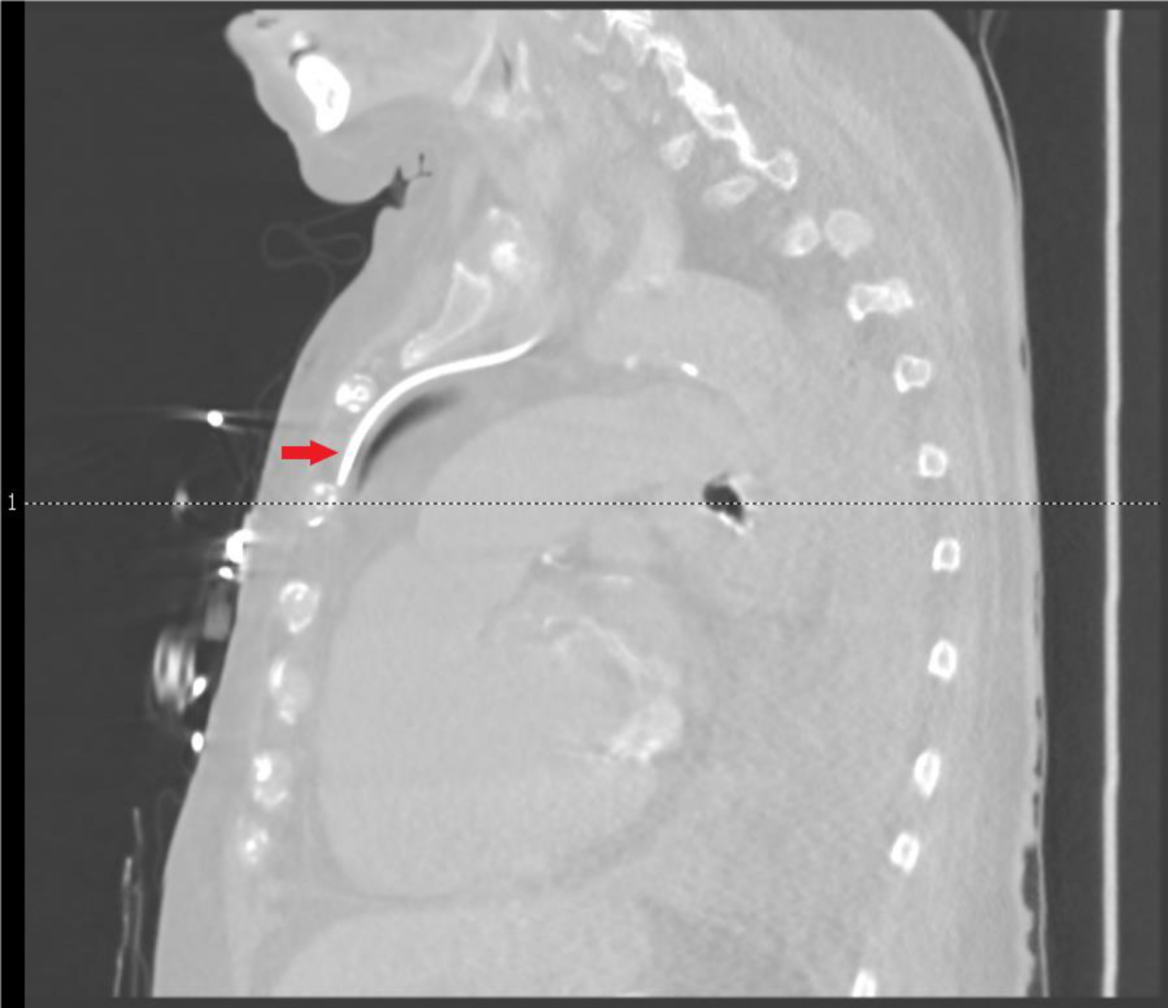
CT chest sagittal view of IJV CVC (red arrow) coursing along the anterior mediastinum, consistent with placement in the left ITV. IJV, internal jugular vein; CVC, central venous catheter; ITV, internal thoracic vein
